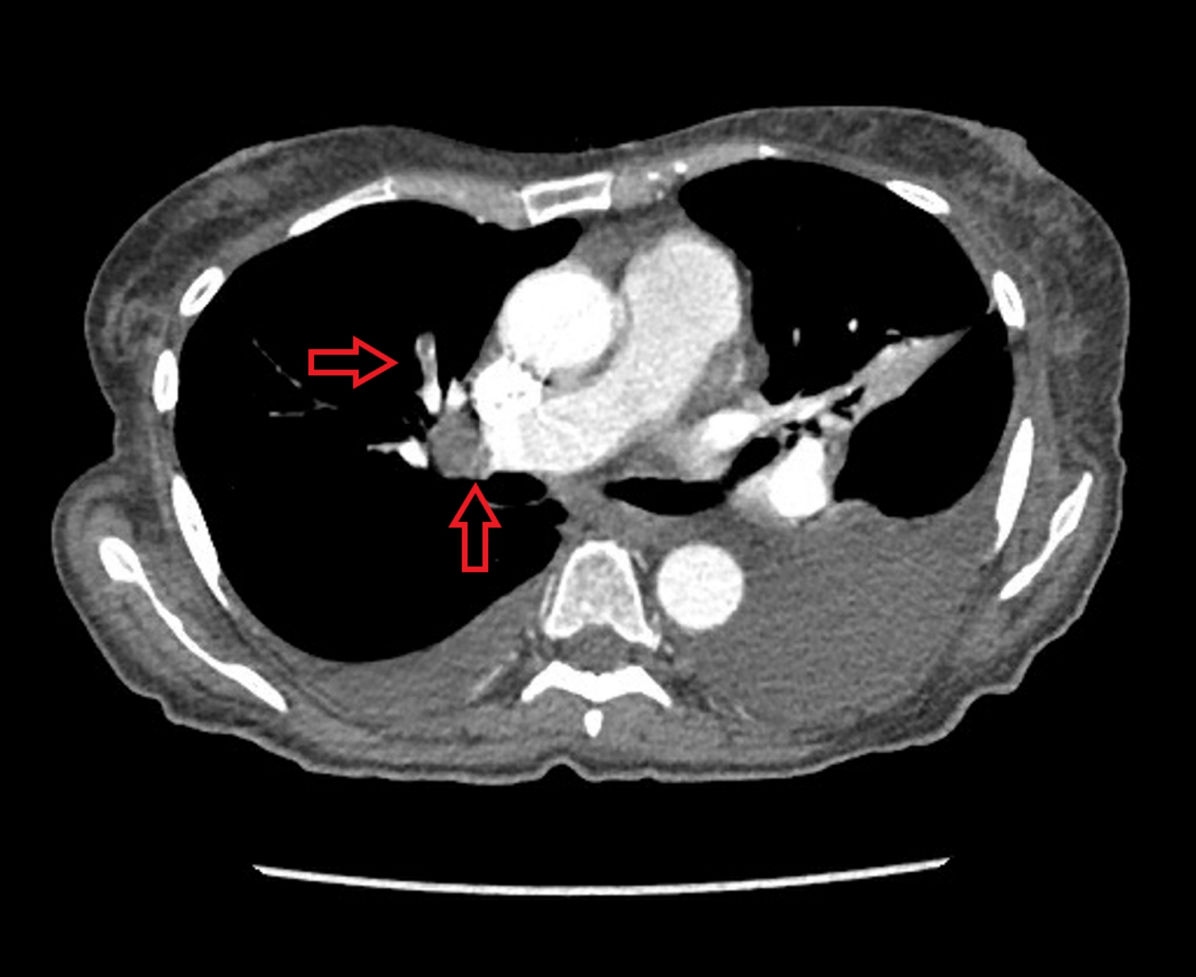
CTA of the chest showed pulmonary emboli throughout the right lung, with the greatest clot burden at the right middle lobe (red arrows) CTA, computed tomography angiogram
